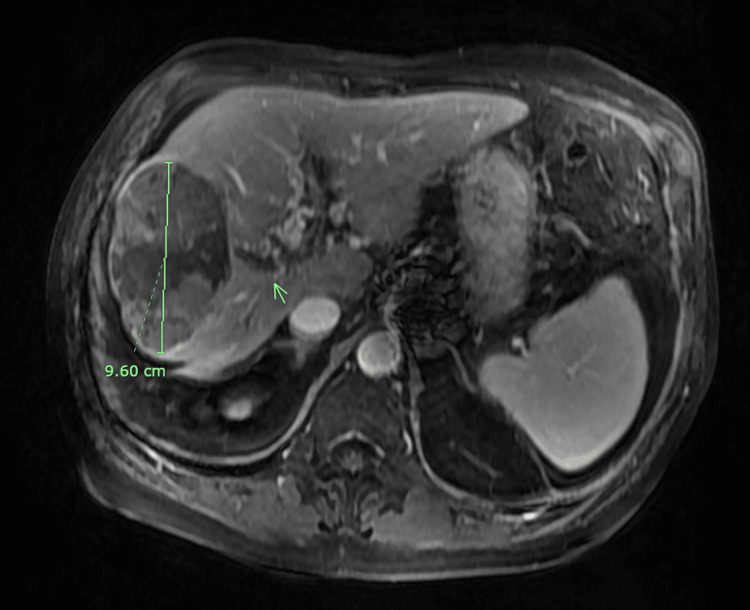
Magnetic resonance imaging of the abdomen with and without contrast transverse section. The green line shows the 9.6 cm hepatocellular carcinoma in the right lobe of the liver. The green arrow points at the hepatocellular carcinoma nodules in the periphery of the large lesion.
